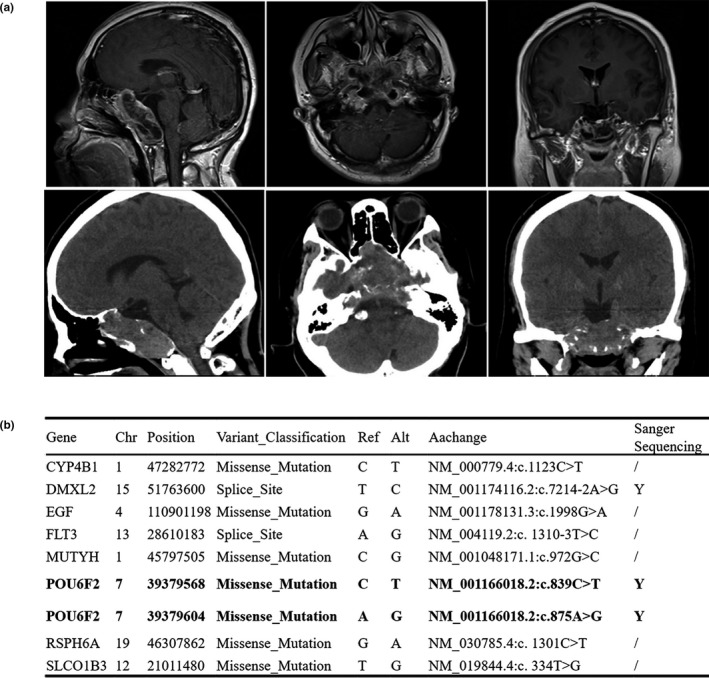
Preoperative images and mutation landscape of the prolactinoma. (a) Preoperative MRI and Computed Tomography (CT) demonstrate a giant adenoma with the invasion of the sphenoid sinus, clivus, and anterior skull base. (b) The mutation landscape of this prolactinoma. MRI, magnetic resonance imaging
